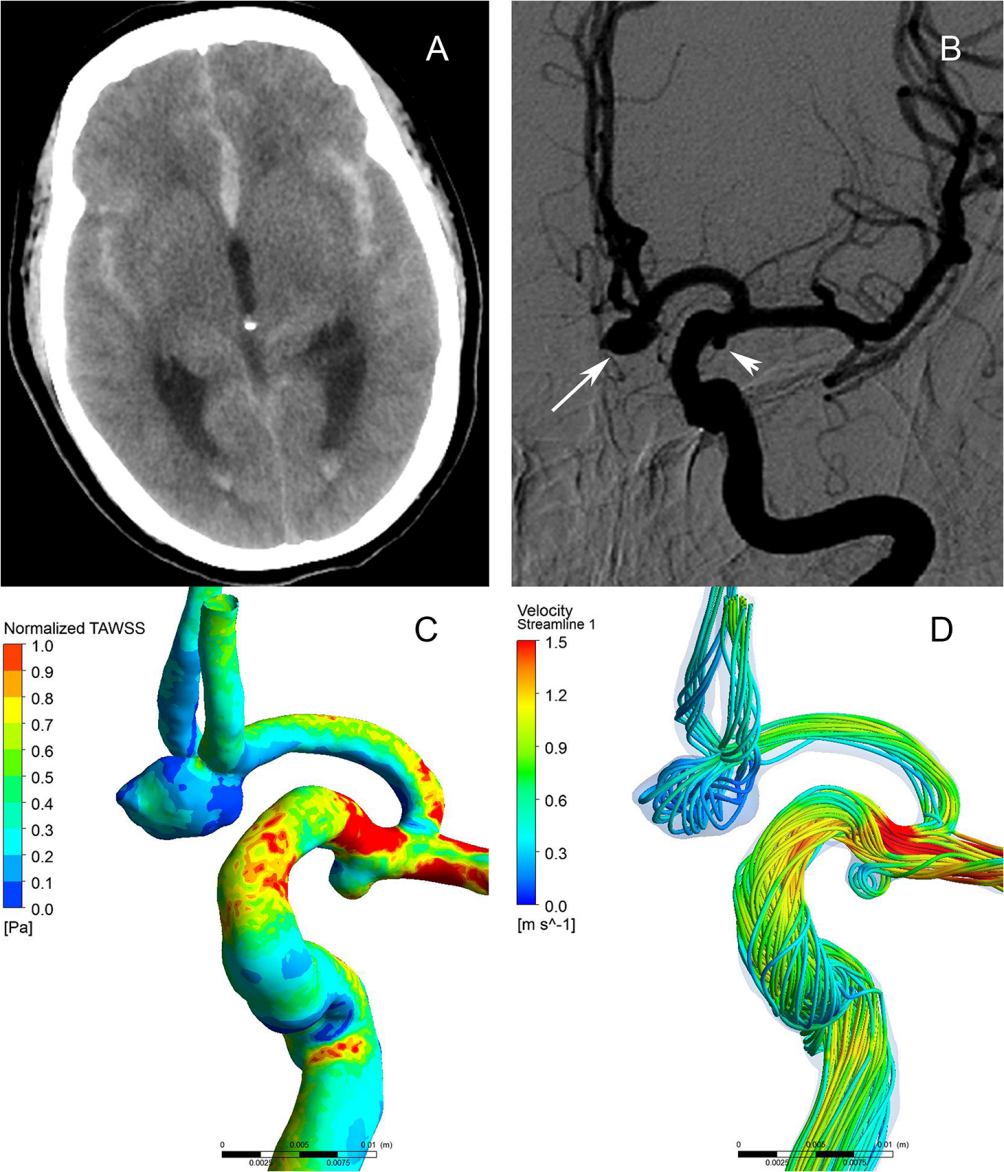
Morphological-hemodynamic characteristics of multiple intracranial aneurysms in the ipsilateral anterior circulation. A 60-year-old female presented with severe headache. CT scan showed subarachnoid hemorrhage (A). The angiograms of left internal carotid artery showed a ruptured aneurysm (B, large arrow) and an unruptured aneurysm (B, small arrow). The distribution of normalized wall shear stress (WSS) magnitude on the aneurysms sac showing the ruptured aneurysm had a lower WSS than the unruptured one (C). The Streamlines showing the flow pattern of the ruptured aneurysm was complex at peak systole (D). Streamlines are rendered with colors according to the velocity magnitude.
